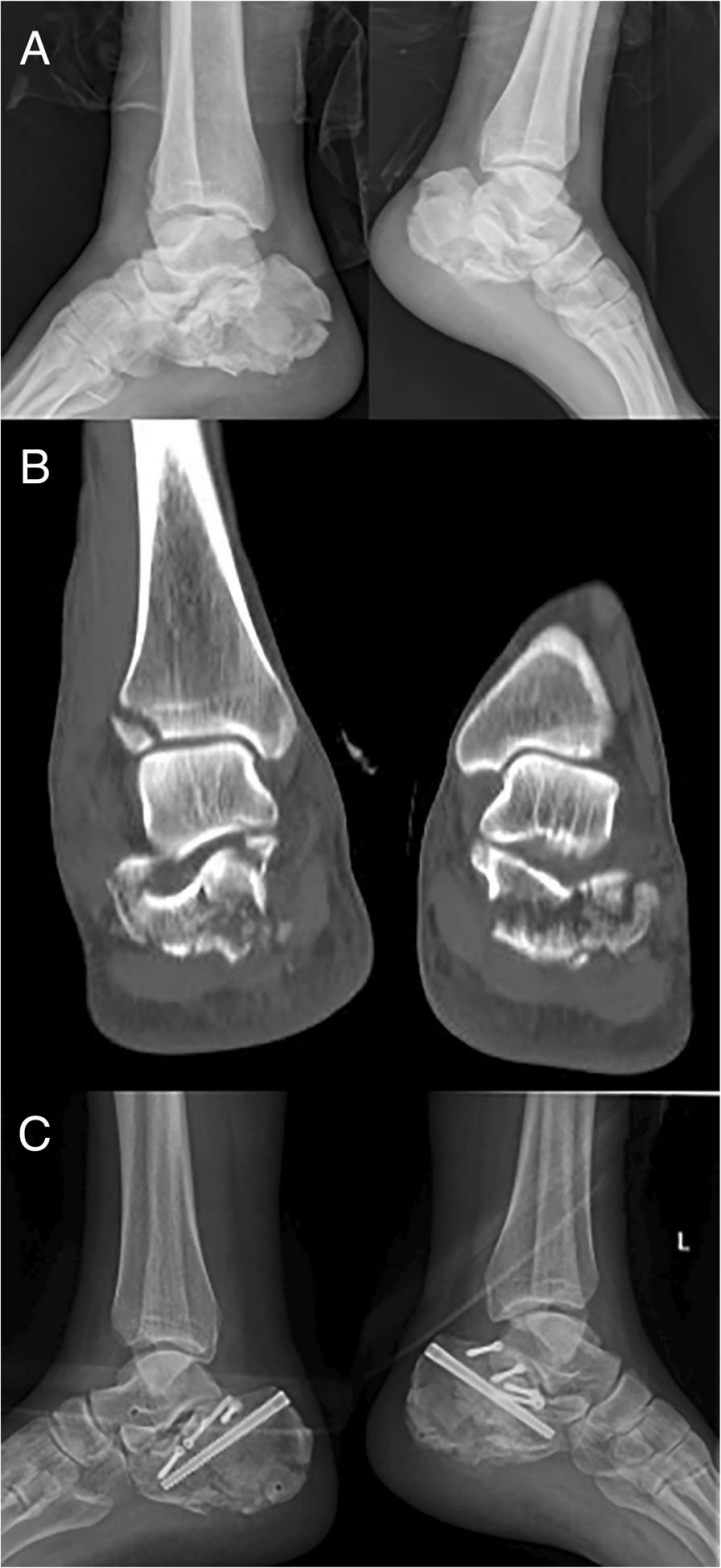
Case 2: A 38-year-old male who was a smoker with bilateral calcaneus fractures. X-ray lateral view (a) and CT scan (b) showing severe compression of the subtalar joint articular surface. Lateral view (c) after surgery showing satisfactory reduction and percutaneous fixations of the calcaneus fracture
